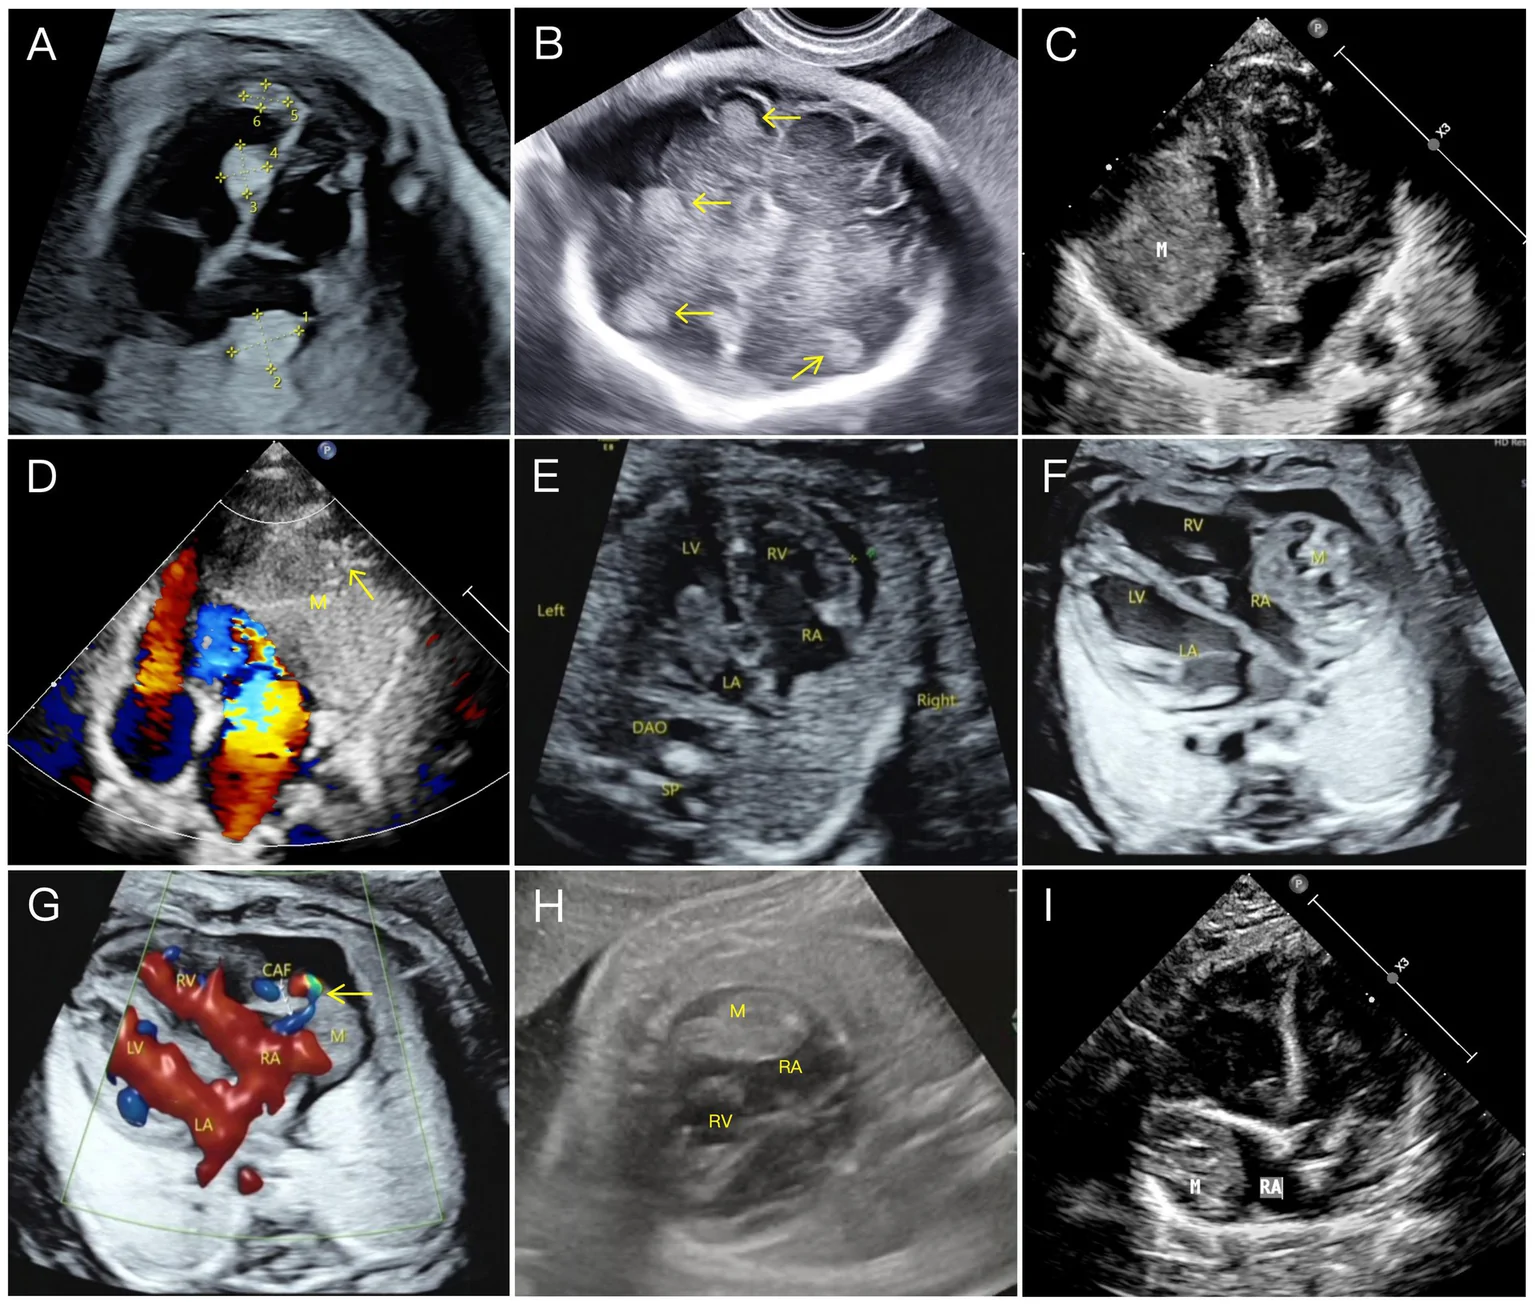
Sonographic features of various cardiac tumors. (A) At 31 + 3 weeks, the fetal heart shows multiple strongly echogenic CRs protruding into the cardiac chambers. (B) Cranial ultrasound of the fetus in (A) reveals multiple parenchymal brain lesions (arrows), suggestive of TSC. (C) A broad-based isoechoic fibroma extending across the left atrium and the basal portion of the left ventricle. (D) Fibroma involving the interventricular septum, apex, and lower lateral wall of the left ventricle, with heterogeneous internal echotexture and visible calcifications (arrow). (E) At 22 + 2 weeks, a myxoma was attached to the annulus of the anterior leaflet of the mitral valve, exhibiting a loose internal echo pattern. (F) At 29 + 1 weeks, a teratoma arising from the right atrial appendage and protruding into the pericardial cavity, presenting as a cystic-solid mass with calcification, accompanied by pericardial effusion. (G) The same case as in (F), complicated by a right coronary artery–right atrial fistula (arrow). (H) At 39 + 3 weeks, a right atrial hemangioma presenting as a slightly hyperechoic mass with small anechoic areas and a broad base. (I) At 45 days after birth, the hemangioma has enlarged and exhibits a spongiform appearance.
